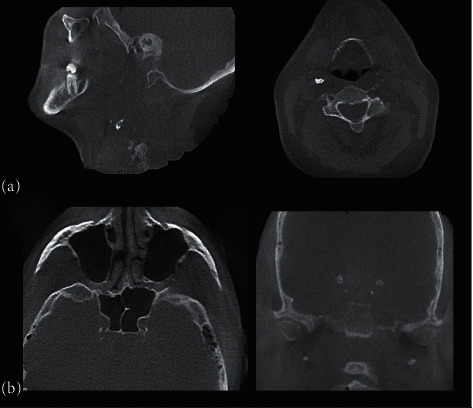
(a) Sagittal and axial CBCT images showing extracranial and (b) axial and coronal CBCT sections showing intracranial carotid artery calcifications.
